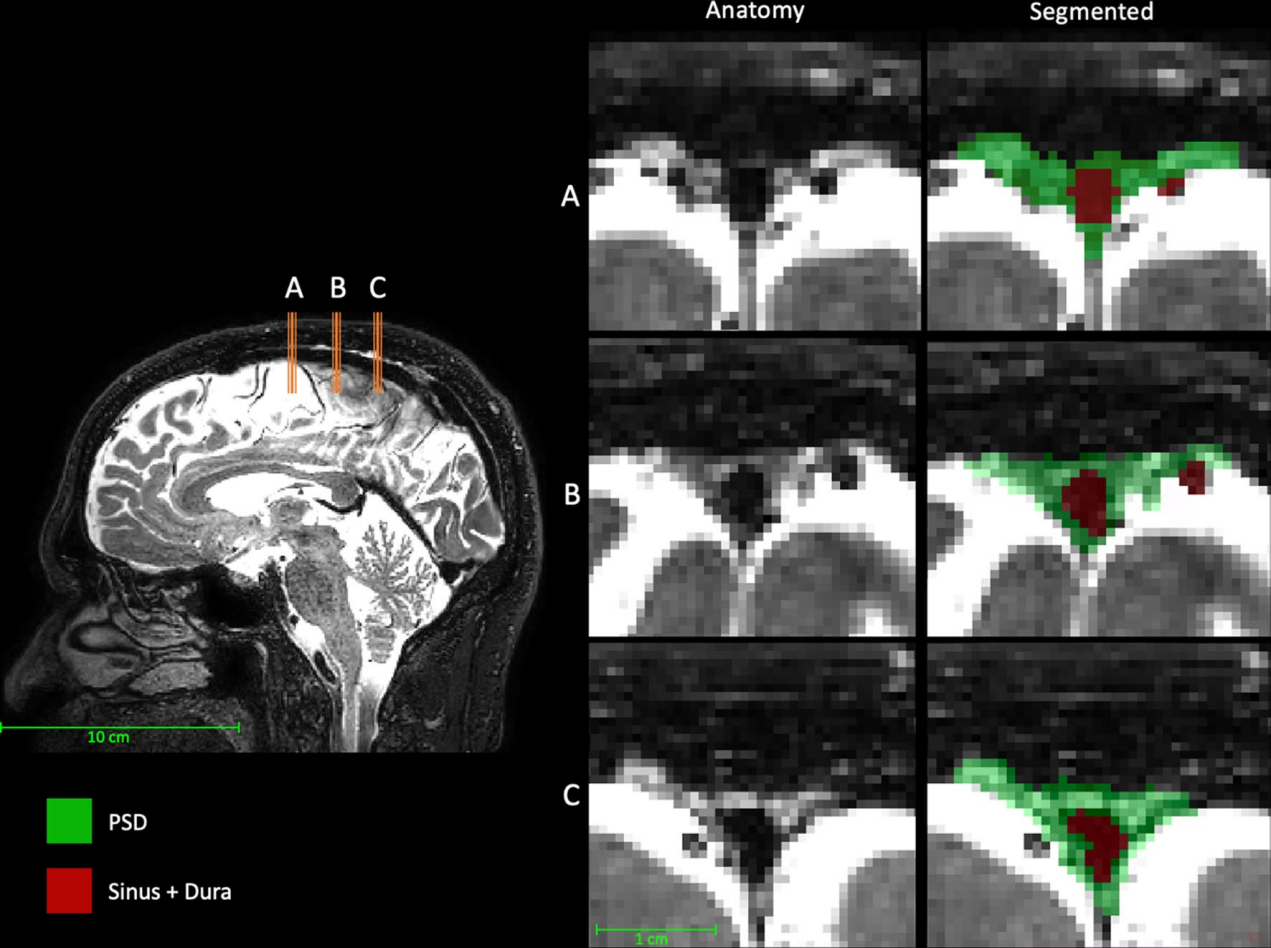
Parasagittal dura space (PSD) definition using *T*_2_-weighted MRI. Three coronal slices are taken from the posterior aspect of the frontal lobe to the medial aspect of the parietal lobe. In this figure the PSD appears in green, the remainder of the parasagittal space appears in red (i.e., sagittal sinus, and afferent veins)
